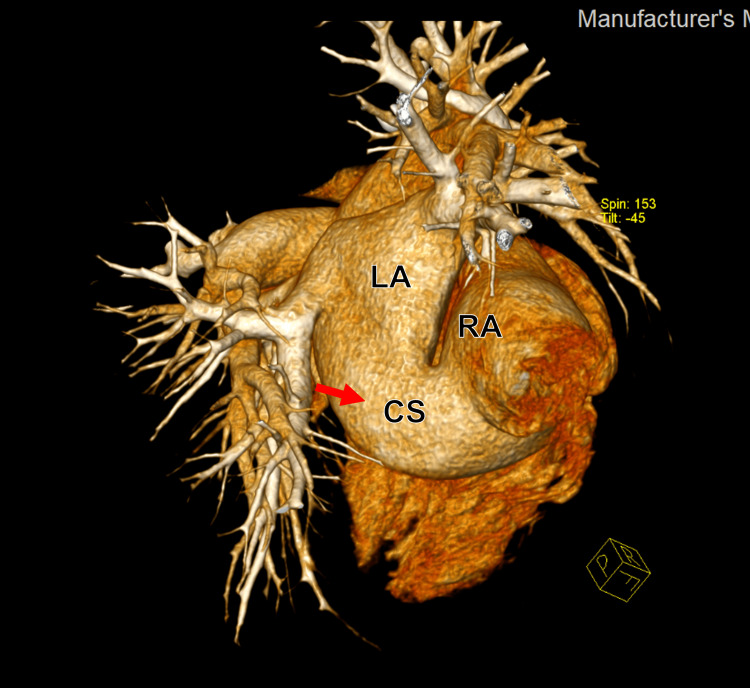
Three-dimensional reconstruction from cardiac computed tomography angiography (CCTA) showing the unroofed and dilated coronary sinus (CS).
